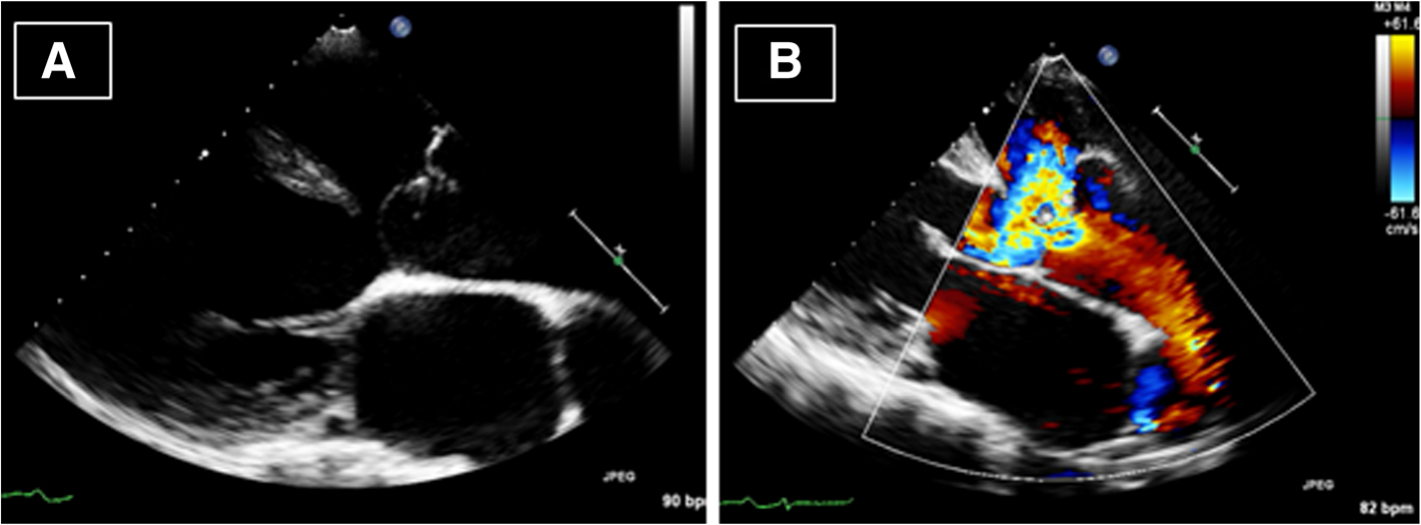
Echocardiogram showing overriding truncus arteriosus (TA), truncus valve (TV) and a large unrestrictive outlet ventricular septal defect (VSD) (a). Color Doppler showing severe truncal valve regurgitation (b)
